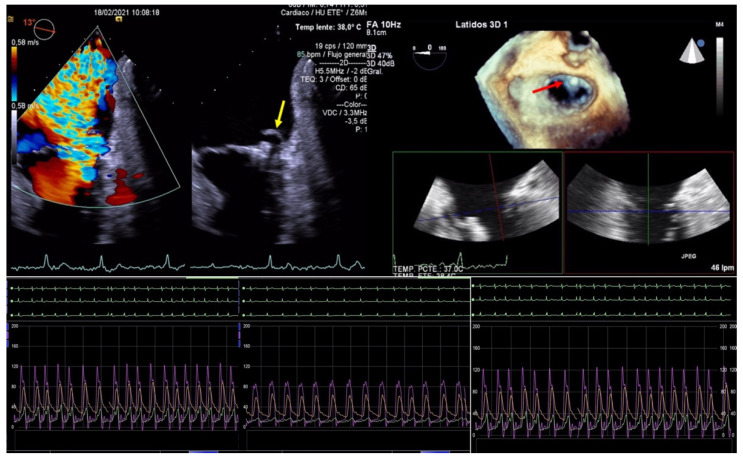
Two-dimensional transesophageal echocardiogram showing a very severe mitral regurgitation after the partial detachment of a clip and a leaflet tear prolapsing in the left atrium (yellow arrow). Three-dimensional transesophageal echocardiogram in the enface mitral view, where a clip can be seen attached to the anterior leaflet (red arrow) and loose from the posterior with a lack of diastolic tissue bridge. In the lower panel, simultaneous recording of left atrium (green), pulmonary artery (yellow), and aortic pressure (purple): baseline, post clip implantation and after detachment.
